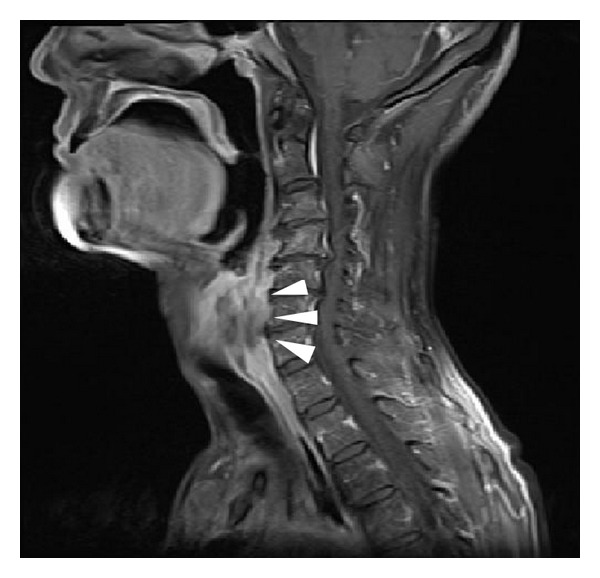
Magnetic resonance T1-weighted, contrast-enhanced image at the first admission. Tumor lesion infiltrated from the posterior wall of hypopharyngeal to the prevertebral muscles.
